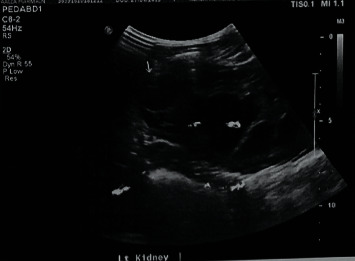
Day 5 post-JJ stenting ultrasound showing resolution of urinoma and decompression of the left kidney.
